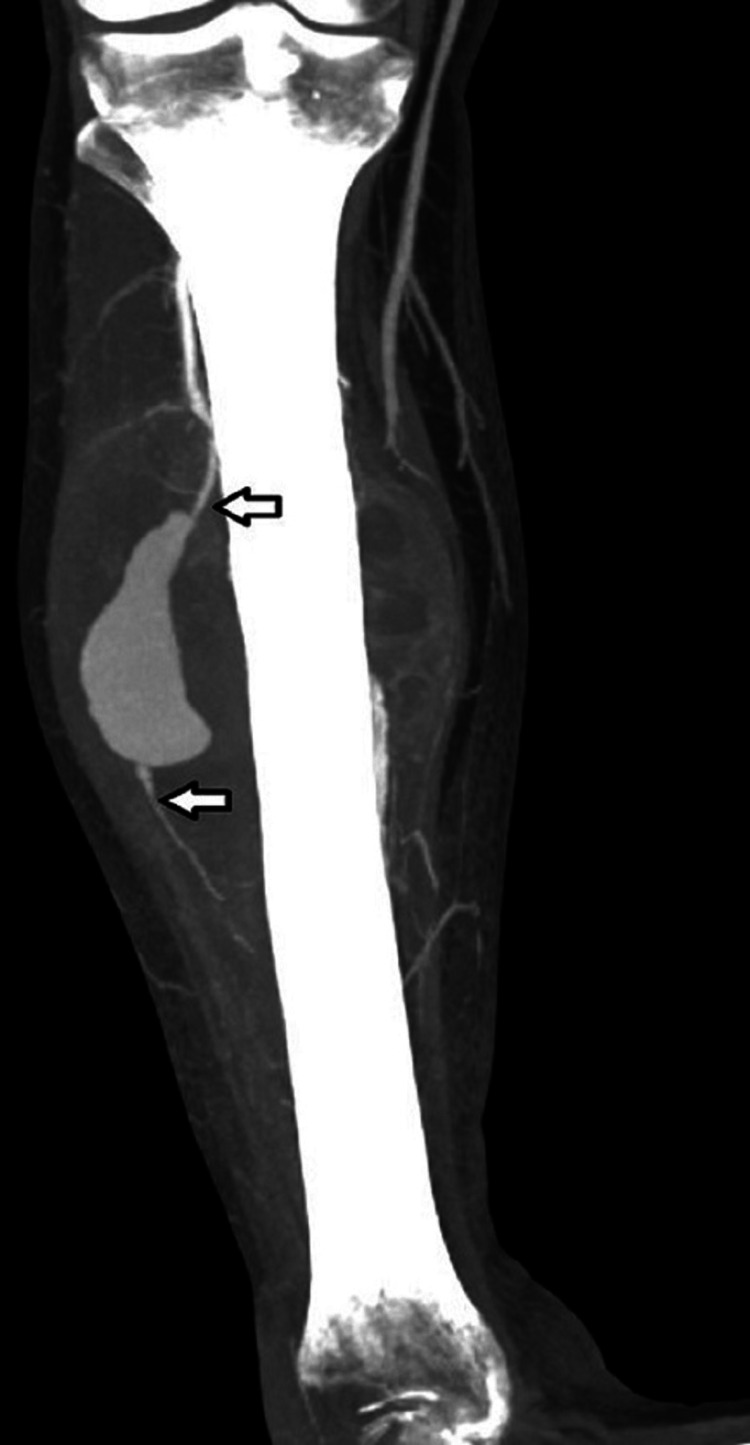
Pseudoaneurysm CT angiography of the right lower extremity confirming the pseudoaneurysm arising from the proximal aspect of the anterior tibial artery (arrows).
